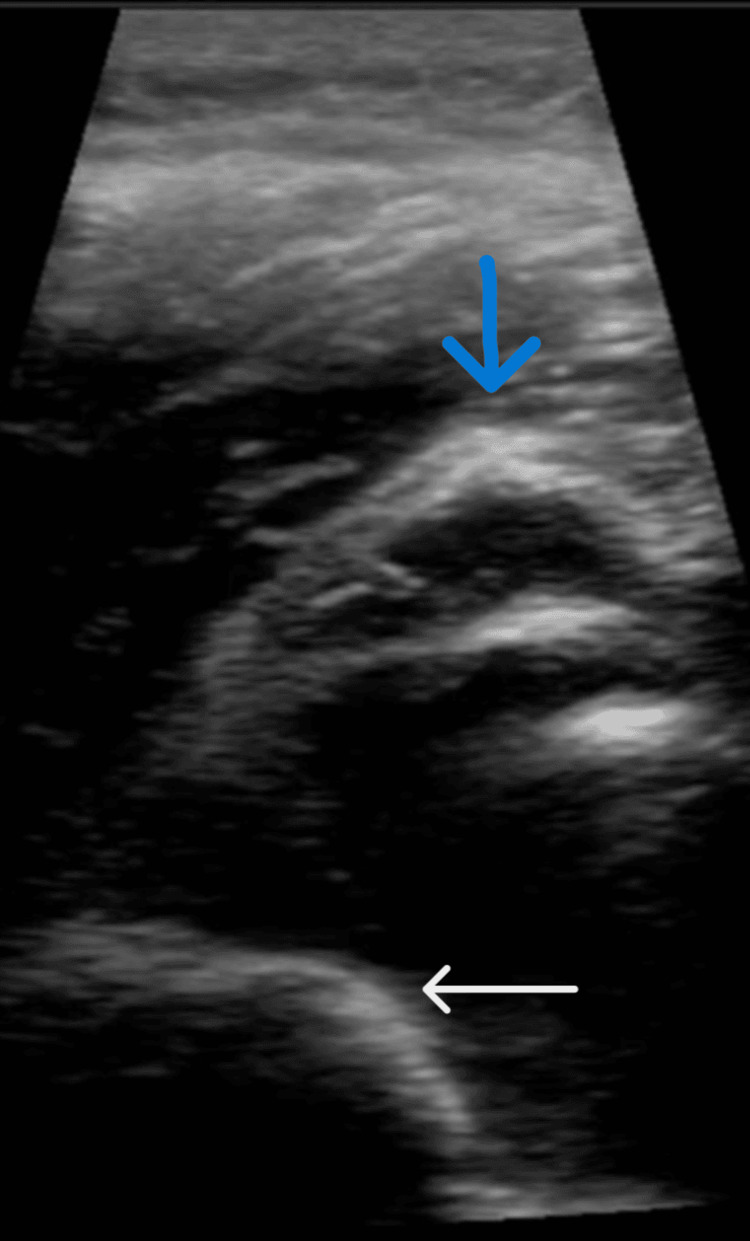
2: Bedside portable ultrasound demonstrating the humeral head (white arrow) anterior to the scapular spine (blue arrow), indicating anterior shoulder dislocation
